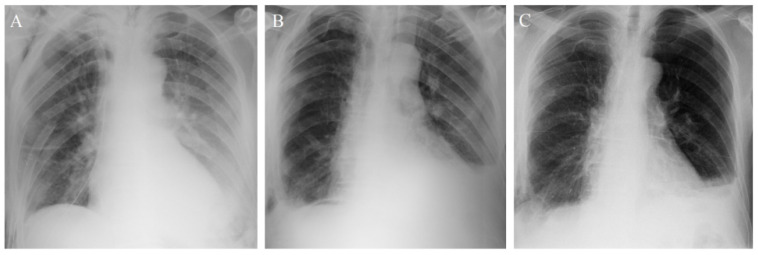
Chest x-rays from post-operative day 2 (A), post-operative day 7 (B), post-operative day 18 (C).
